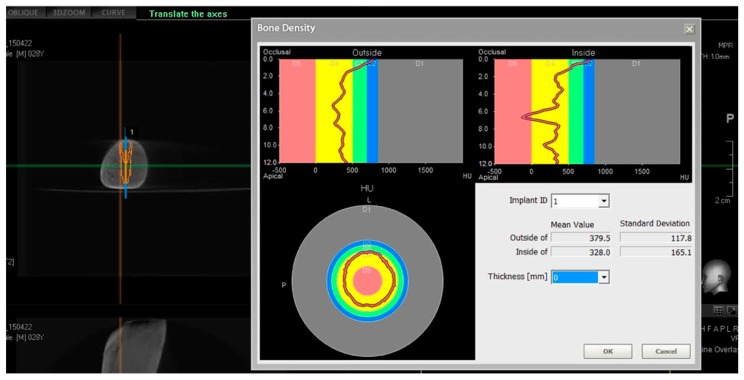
Scanning of the ribs with CBCT scan (Pax i-3d Green, Vatech, Yongin, Korea) to evaluate bone density and select implant site, using Hounsfield measurement unit (Density of 750 < HU).
